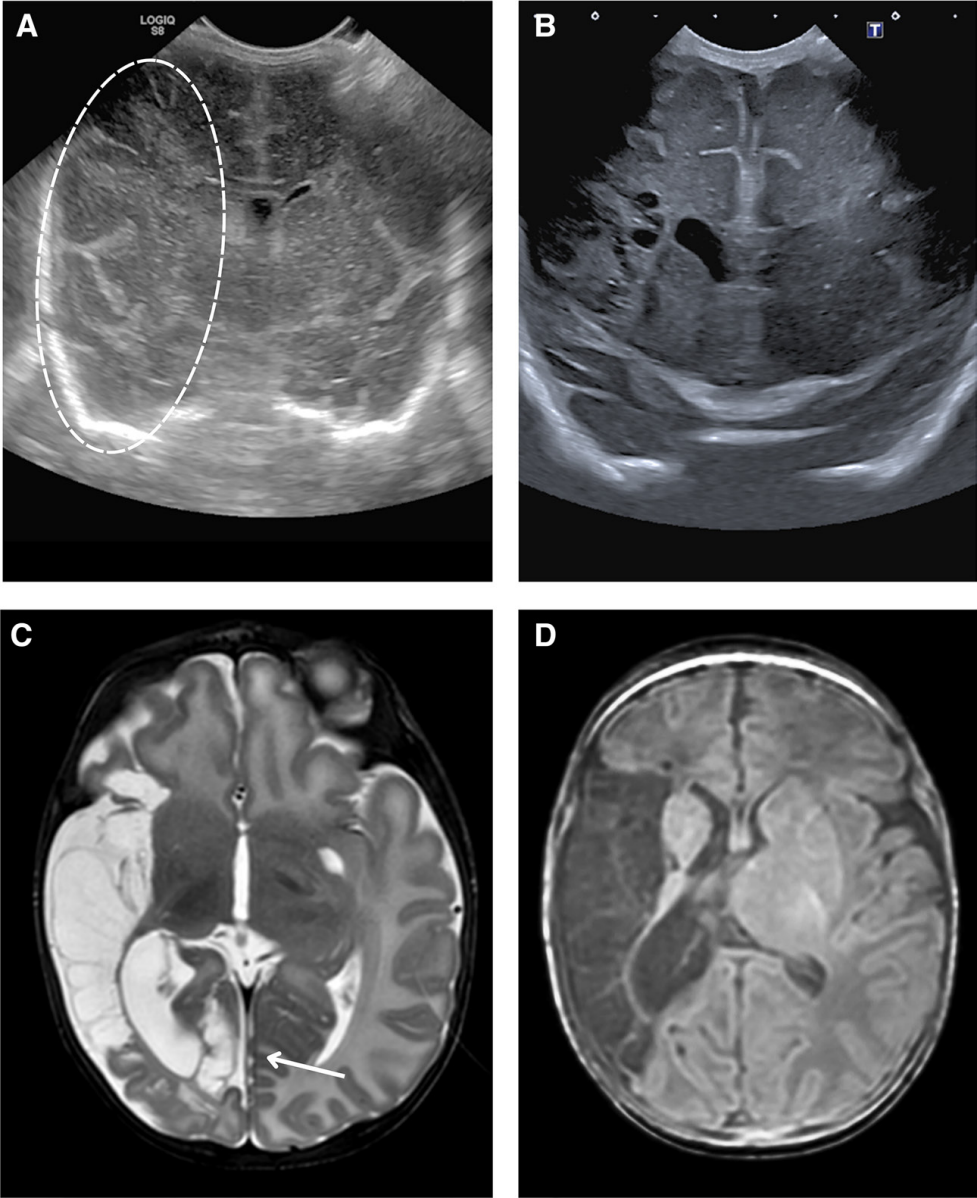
Case of postnatal arterial ischemic stroke involving both right main branch middle cerebral artery (MCA) and posterior cerebral artery (PCA), in an uncomplicated twin pregnancy, with a second necrotizing enterocolitis surgery at postnatal 94 (twin 18). A, Coronal cranial ultrasound (cUS) on postnatal day 95 demonstrating a demarcation line surrounding the right insula (encircled) and compression of the right lateral ventricle. B, Coronal cUS on postnatal day 134, revealing cystic lesions within the right hemisphere parenchyma and subsequent right lateral ventriculomegaly. C, Axial T2-weighted magnetic resonance imaging (MRI) at term-equivalent age confirming a right MCA territory stroke, also involving the PCA territory (arrow), with involvement of the right optic radiation. D, Axial T1-weighted MRI at the same time point demonstrating asymmetrical posterior limb of the internal capsule development. Although the infant was not yet seen at follow-up, these findings are consistent with a predicted outcome of left-sided hemiparesis, with potential sequelae including epilepsy and left-sided visual field deficits (Table S4—twin 18).
